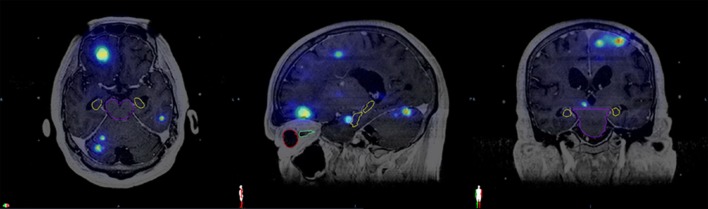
Fused magnetic resonance imaging from the same patient with cumulative isodose distribution displayed in the axial, sagittal, and coronal views along with contours of various organs at risk. Brainstem (purple), hippocampus (yellow), right eye (red), right optic nerve (green).
